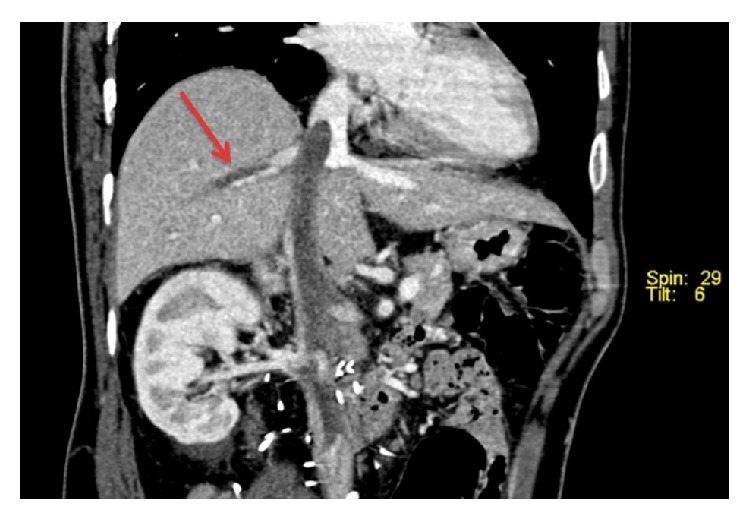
Reformatted coronal CT image demonstrating tumour thrombus in the inferior vena cava, extending into the right hepatic vein.
